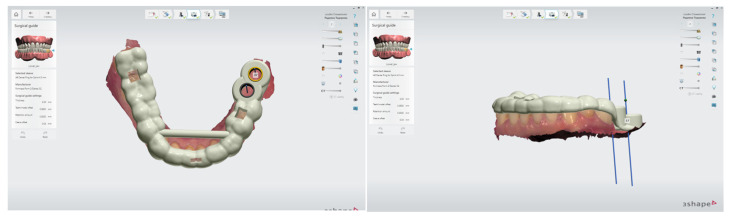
Static surgical guide designed for guided implant surgery. The guide is digitally planned based on CBCT data and intraoral scans, allowing for precise three-dimensional positioning of the implants according to the prosthetically driven treatment plan. 3Shape Implant Studio. CAD/CAM Center, Faculty of Dental Medicine, Medical University of Plovdiv.
